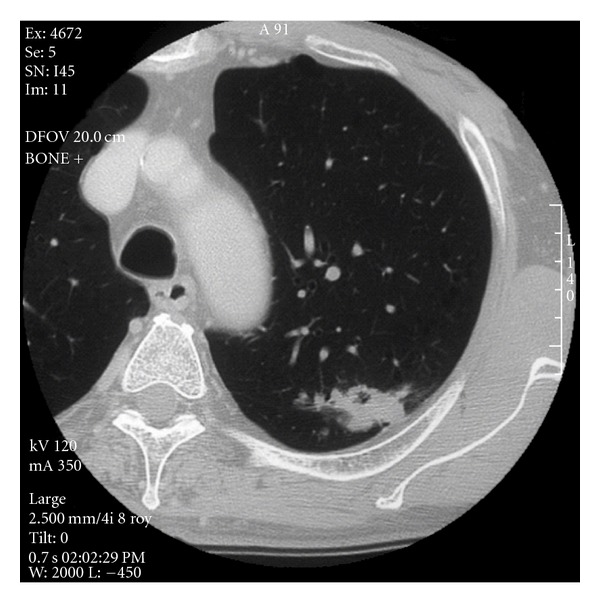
CT scanning of chest showing a lung cancer of 33 mm in the left S^1+2^.
